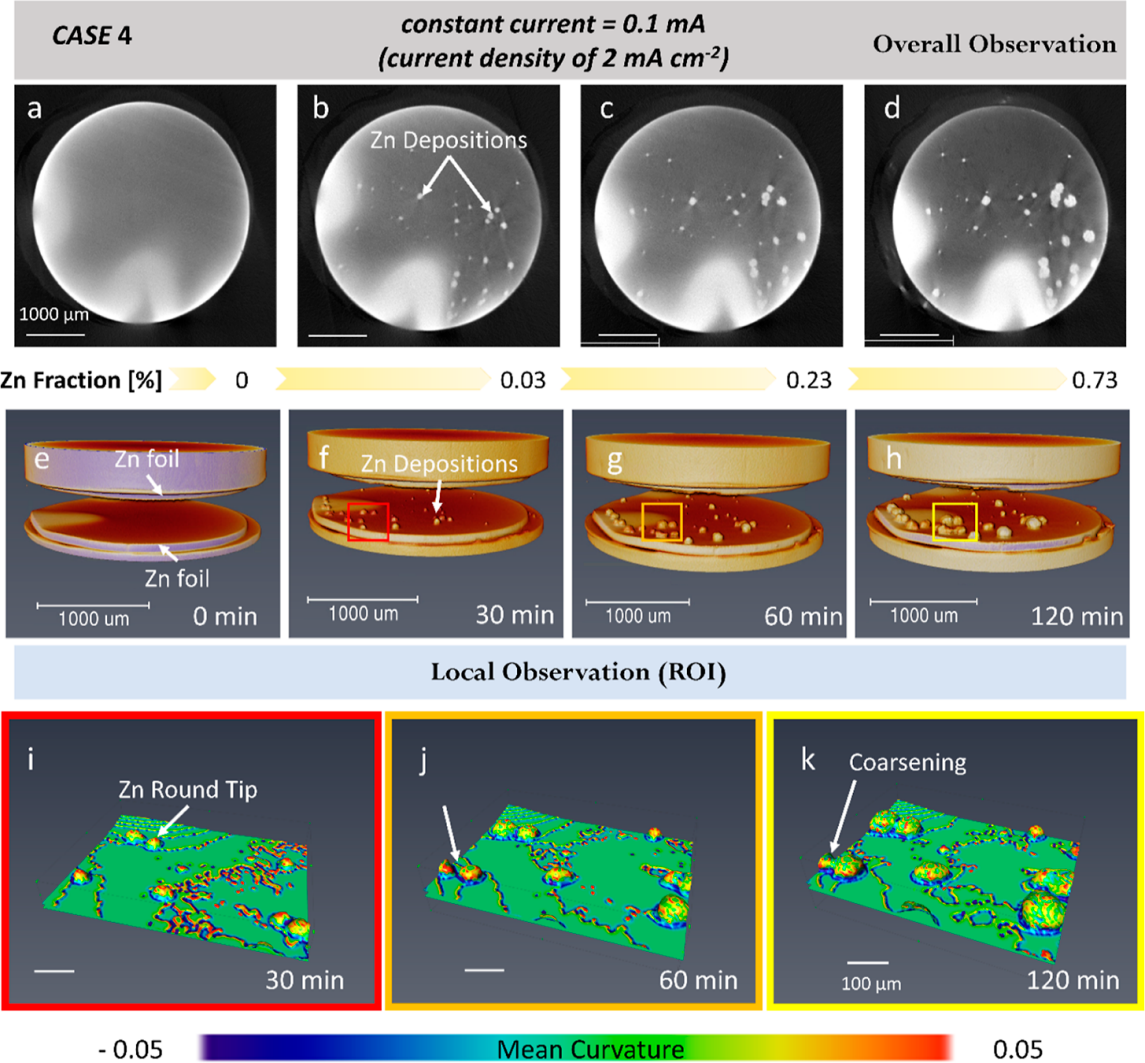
4D X-ray tomographic imaging of the slow zinc depositions
